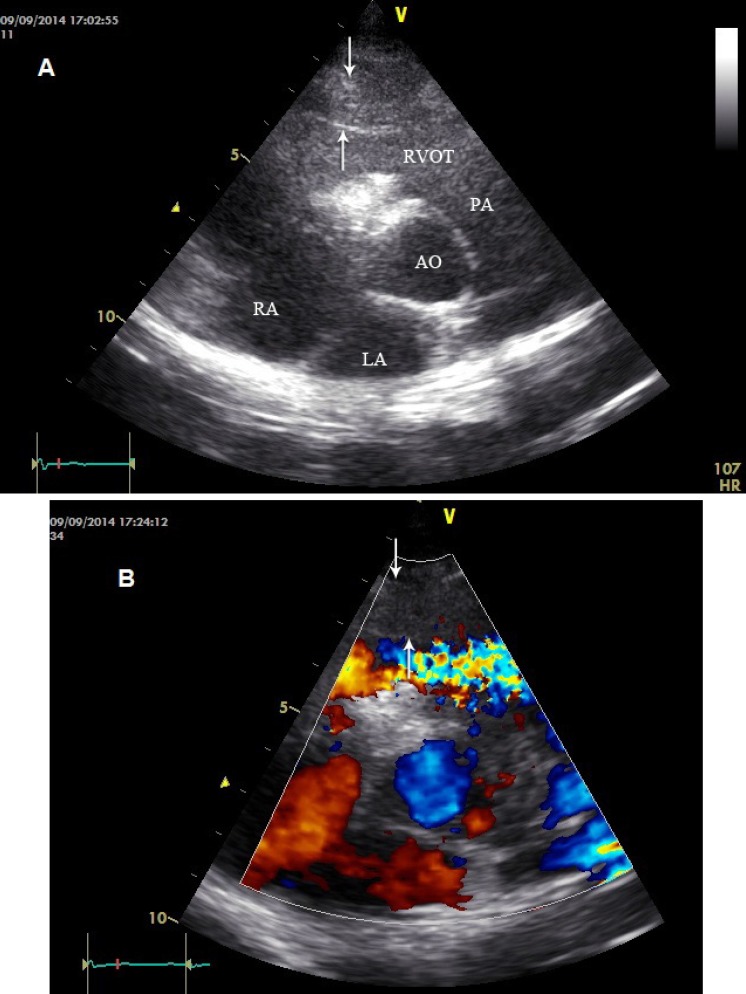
A) Hypertrophy (between two arrows) of the right ventricular outflow tract (RVOT) anterior wall in the parasternal short-axis view at the level of the aortic valve and the ventricular septal defect (VSD) device occluder in two-dimensional echocardiography. B) Turbulent flow at the RVOT at the VSD device site in color-flow Doppler in the parasternal short-axis view at the level of the aortic valve
